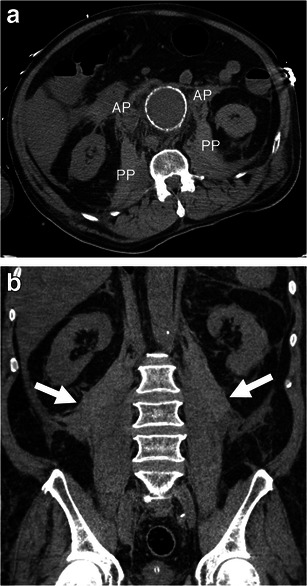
Retroperitoneal haematoma. a Axial unenhanced CT image of a 72-year-old man demonstrates haemorrhage involving bilateral anterior (*AP*) and posterior (*PP*) pararenal spaces as well as the area along the psoas muscles. The left kidney is displaced laterally. Attenuation of 45 HU (more than 30 HU) indicates acute haematoma. b Coronal unenhanced CT image of the same patient shows bilateral retroperitoneal haematomas (*white arrows)*
